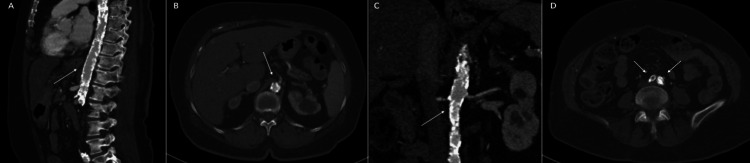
Pre-operative computed tomography angiogram (CTA) images of the aorta. (A) Sagittal view showing dense circumferential calcifications of the thoracic and abdominal aorta with areas of luminal compromise (white arrow). (B) Coronal view revealing similar calcifications and luminal narrowing (white arrow). (C) Severe calcific disease of the supra-celiac aorta (white arrow). (D) Significant narrowing of the aortic bifurcation and common iliac arteries (white arrow).
